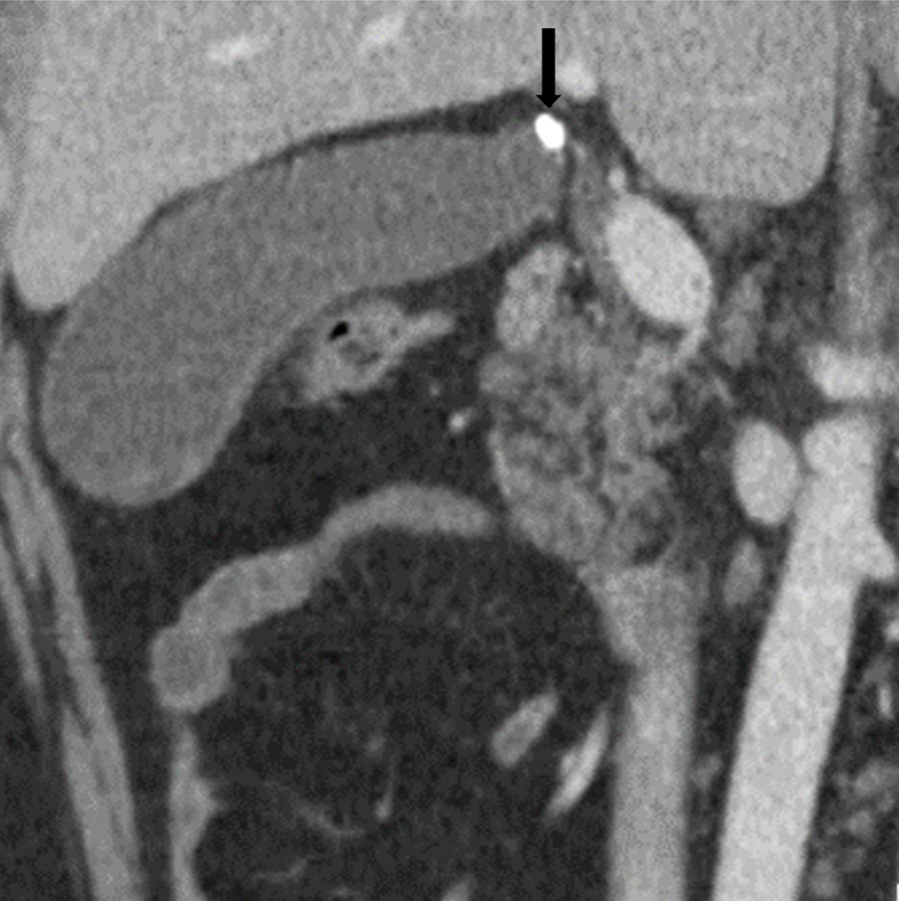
Computed tomography. Arrow indicates incarceration of a small in the neck of the gallbladder
